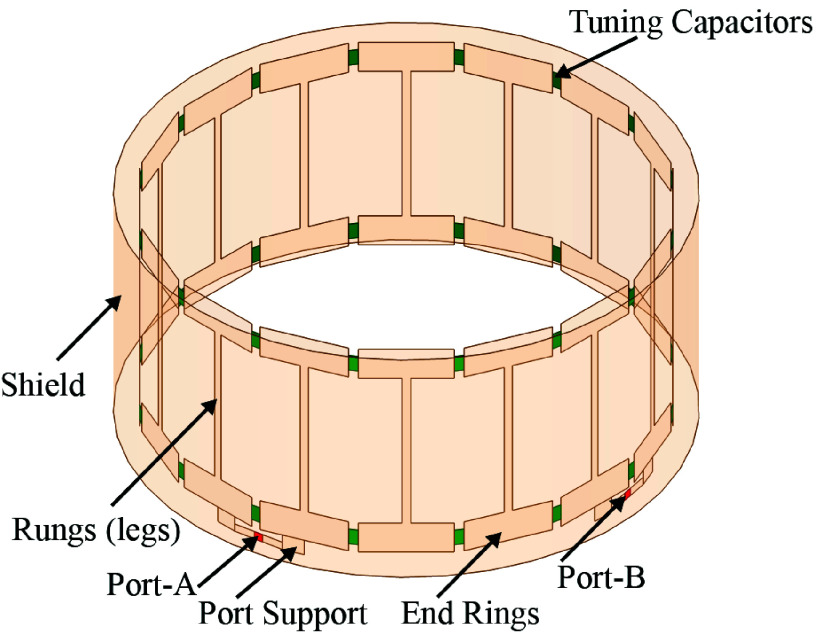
Typical MRI Birdcage coil in high-pass configuration includes 16 rungs and 32 tuning capacitors, with two ports positioned at a 90° displacement from each other.
